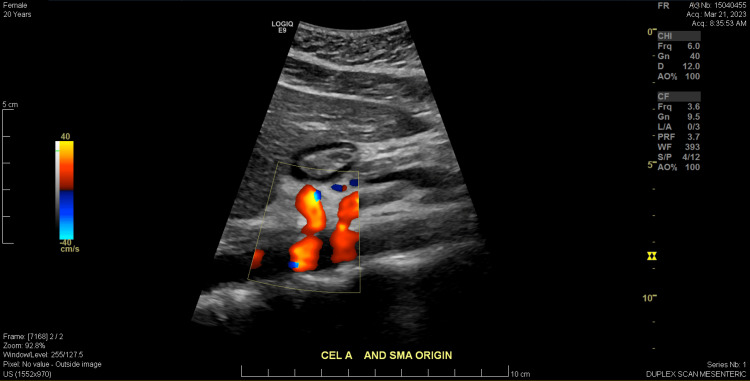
Patient’s abdominal ultrasound capturing the celiac artery and superior mesenteric artery origins. Color Doppler was used to assess for possible stenosis on visual examination, which raised concern for arterial narrowing and prompted further evaluation with flow velocities.
